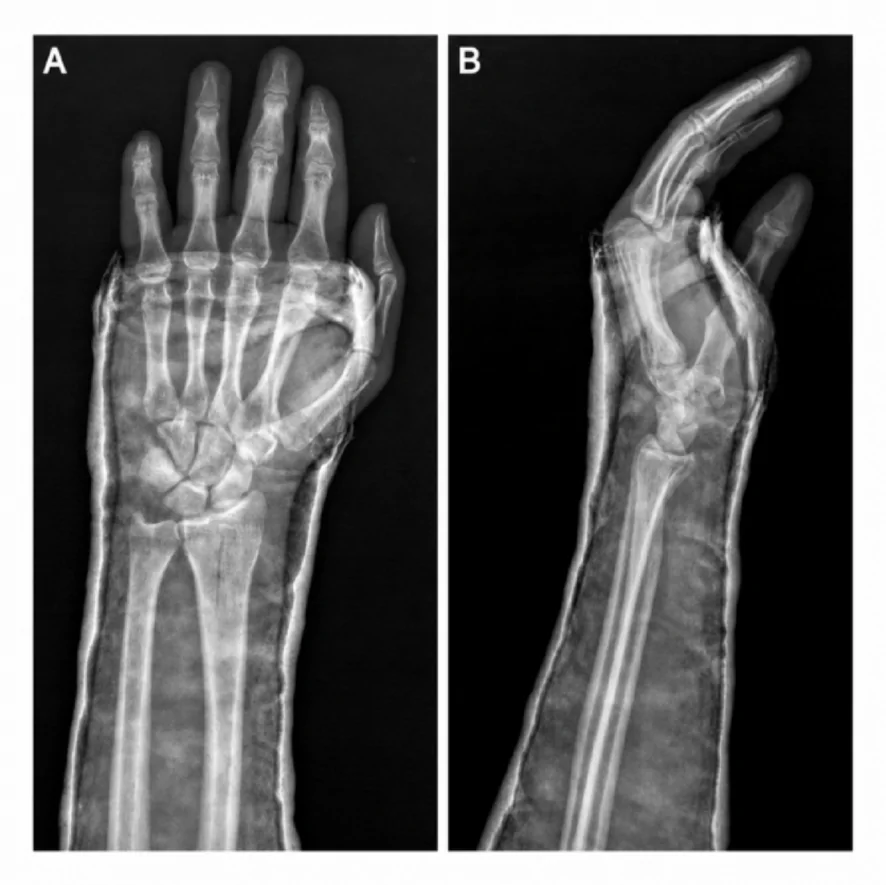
Post-reduction posteroanterior (A) and lateral (B) radiographs of an AO type B distal radius fracture following closed reduction and immobilization
